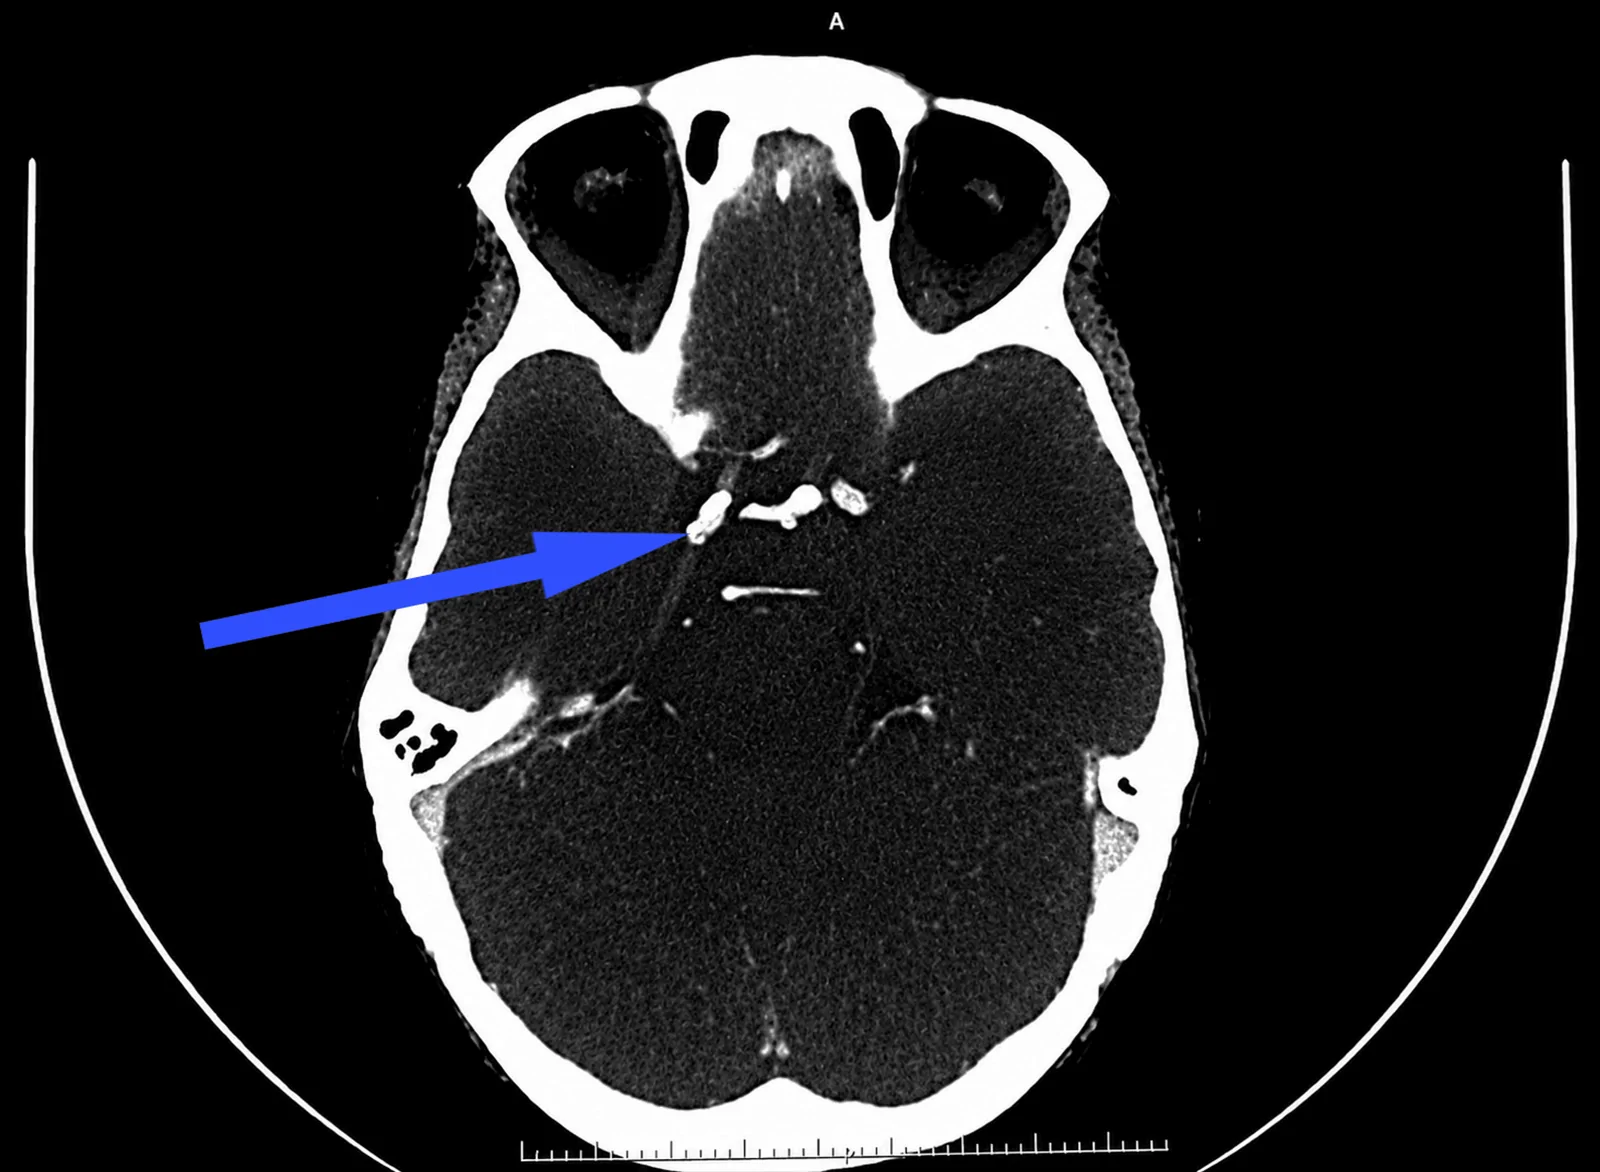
Carotid and cerebral computed tomographic angiography Thick eccentric wall calcification with severe stenosis in the right supraclinoid segment (blue arrow).
